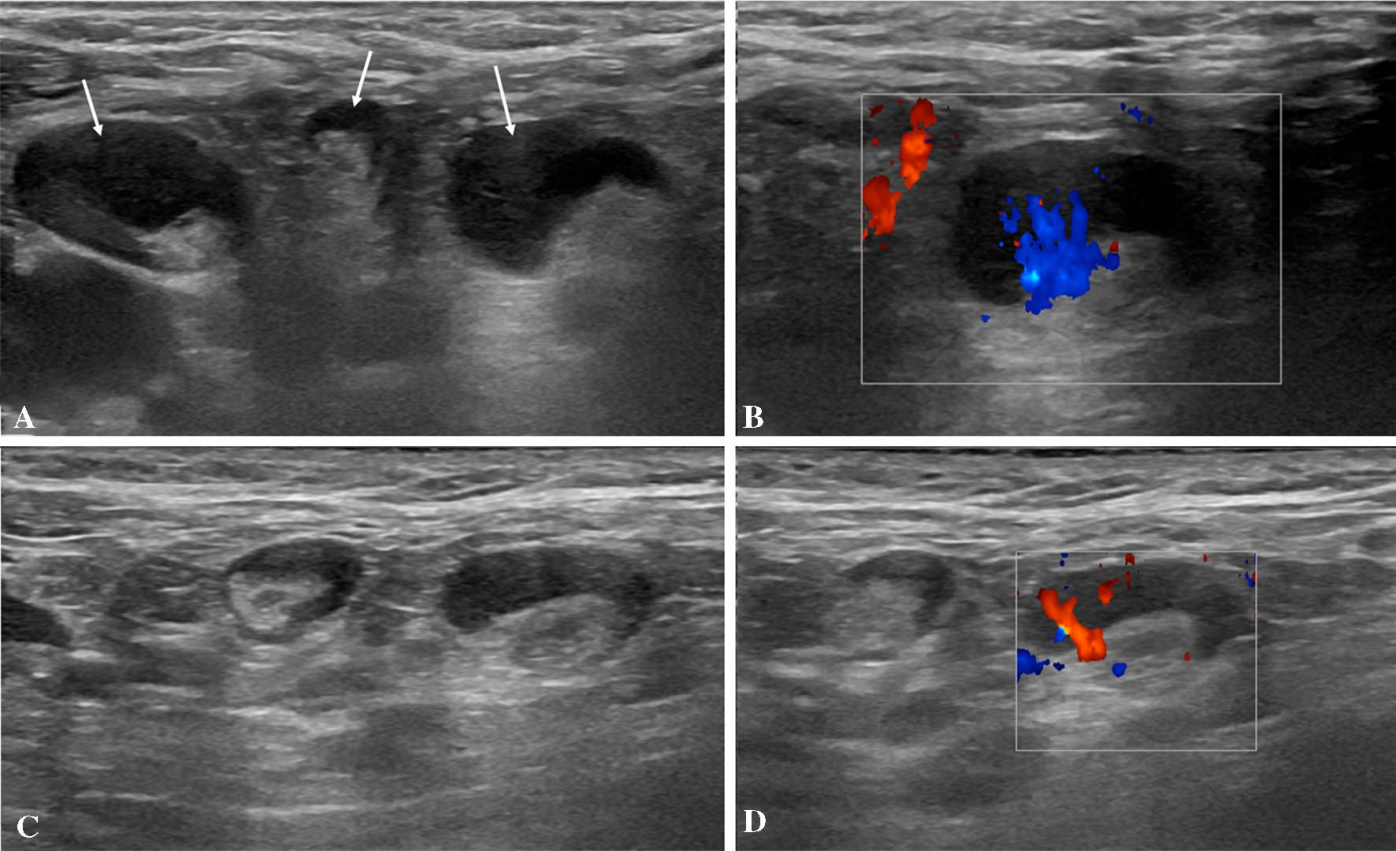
B-Mode (A) and color-Doppler (B) US examination performed in a 28-year-old woman 2 days after receiving the second dose of vaccine: axillary lymph nodes (maximum diameter 14 × 9 mm) with fatty hilum but diffuse cortical thickening (maximum 10 mm, arrows in A) and vivid hilar and cortical vascularization (B) were detected. B-Mode (C) and color-Doppler (D) US examination performed after 4 weeks: reduction of lymph nodes size (7 × 6 mm), cortical thickness (2.9 mm) and vascularization was observed
